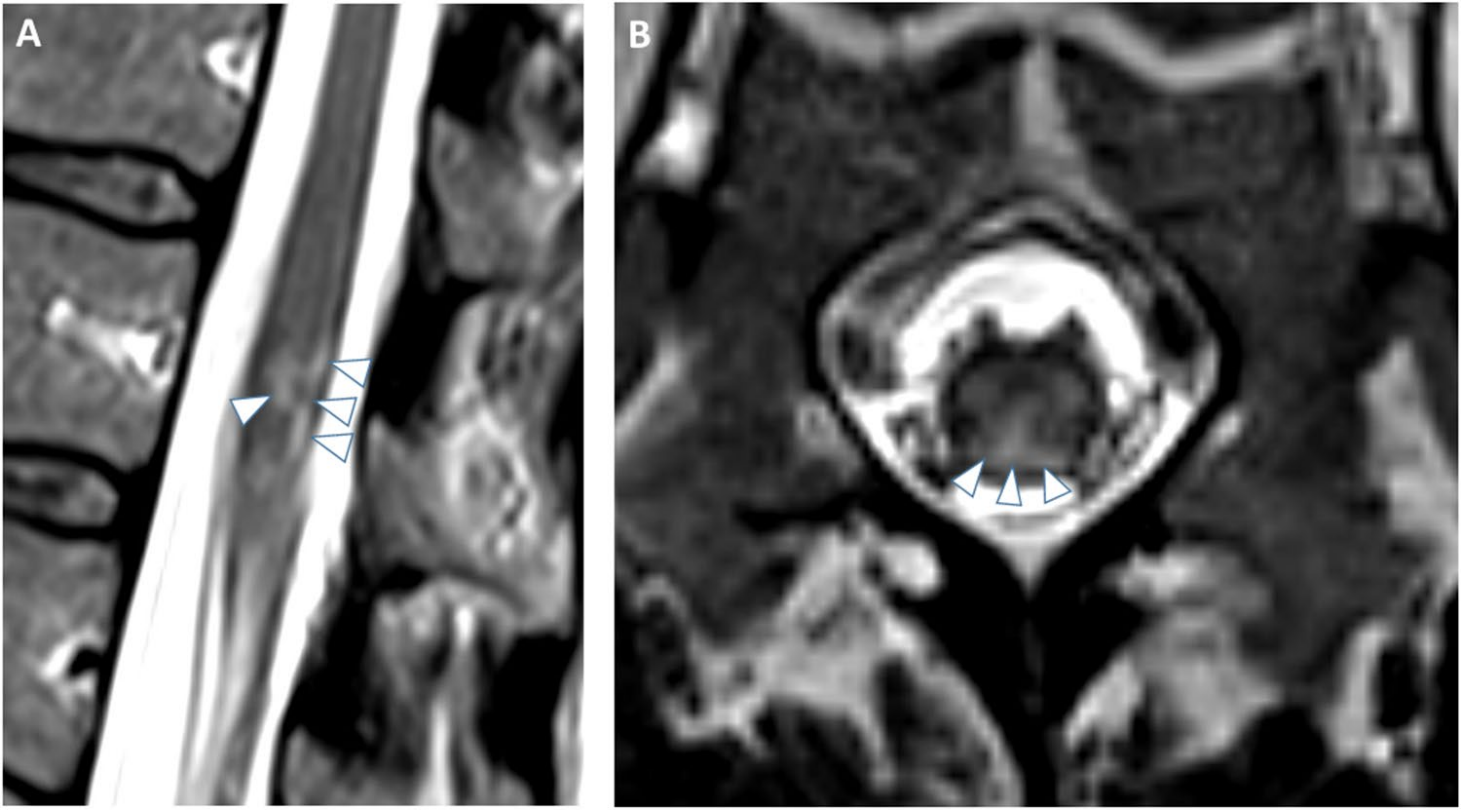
T2-weighted imaging in sagittal (A) and transversal (B) planes reveal bilateral hyperintensities (arrowheads) affecting the medullary gray matter at the conus level, predominantly affecting the posterior portion
